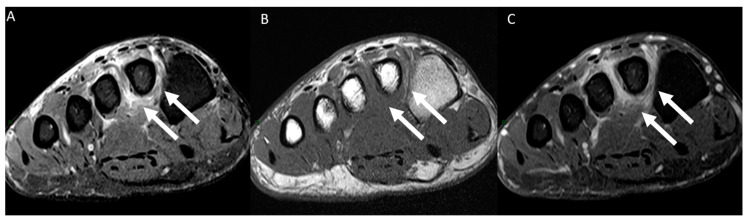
A 36-year-old man presented with right foot pain persisting for 2 days. Forefoot MRI images in the short-axis plane, including a T2-weighted fat-suppressed scan (A), a T1-weighted scan (B), and a post-gadolinium T1-weighted fat-suppressed scan (C), revealed thrombosis in the communicating veins (arrows). This thrombosis was characterized by venous dilation, intraluminal thrombus and an intravenous filling defect.
